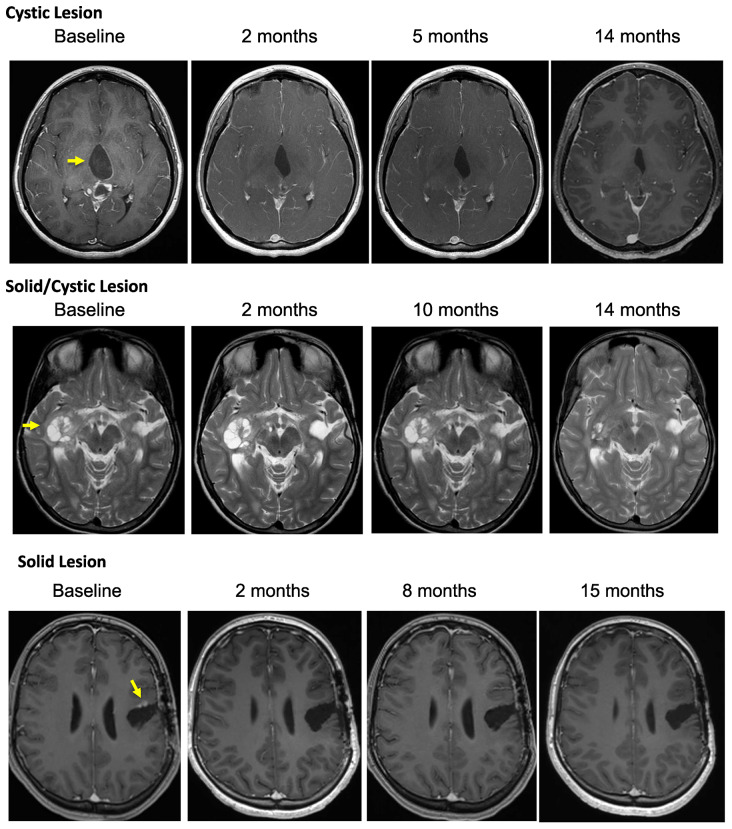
Depicted are representative images of subjects treated on PNOC-002 demonstrating. (A) regression of a contrast enhancing cystic lesion on a contrast, T1 weighted MR image over time; (B) regression of a solid/cystic lesion on a T2 weighted MR image over time; (C) regression of a contrast enhancing solid lesion on a contrast, T1 weighted MR image over time.
